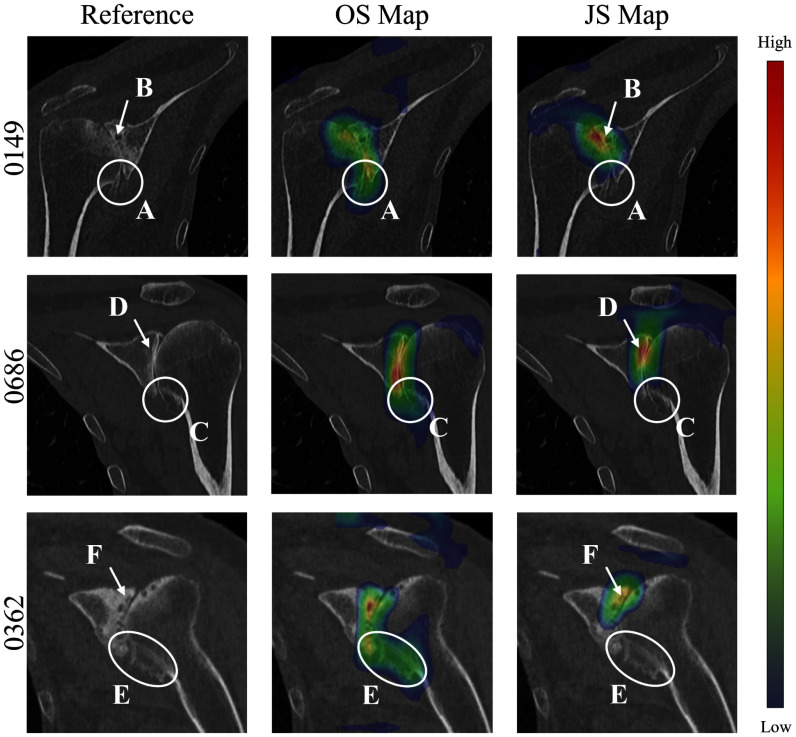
OS and JS GradCAM-generated activation maps (second and third column, respectively) overlaid on top of their corresponding input CT (coronal view). A, C, and E areas highlight large humeral osteophytes, while B, D, and F arrows the non-detectable joint space between the humerus and the glenoid.
